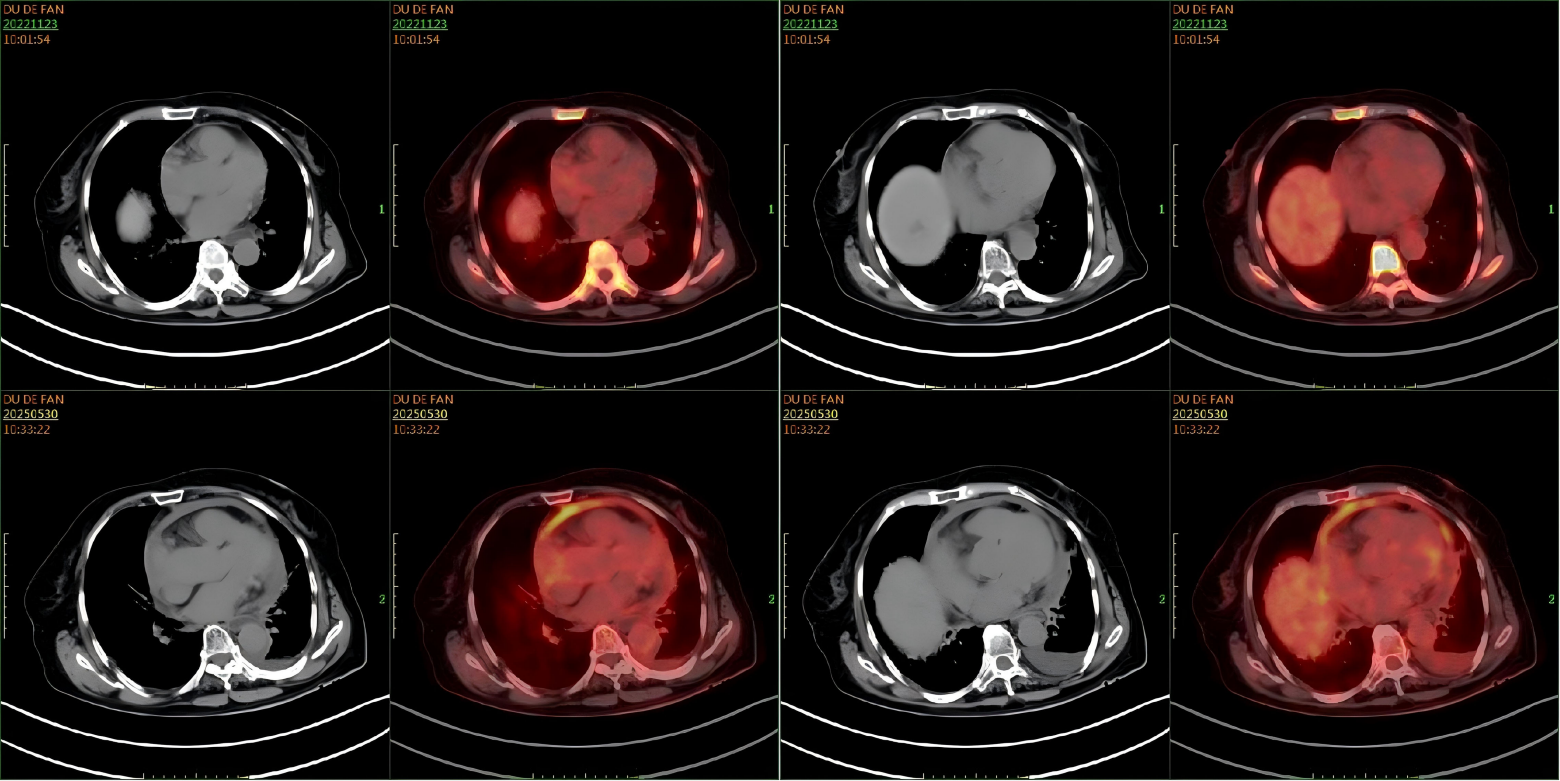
Comparison of PET-CT scans. A comparison of cardiac imaging from November 23, 2022, and May 29, 2025, reveals new findings of pericardial thickening with decreased fluid density, cardiac enlargement, and increased peripheral fluorodeoxyglucose (FDG) uptake.
